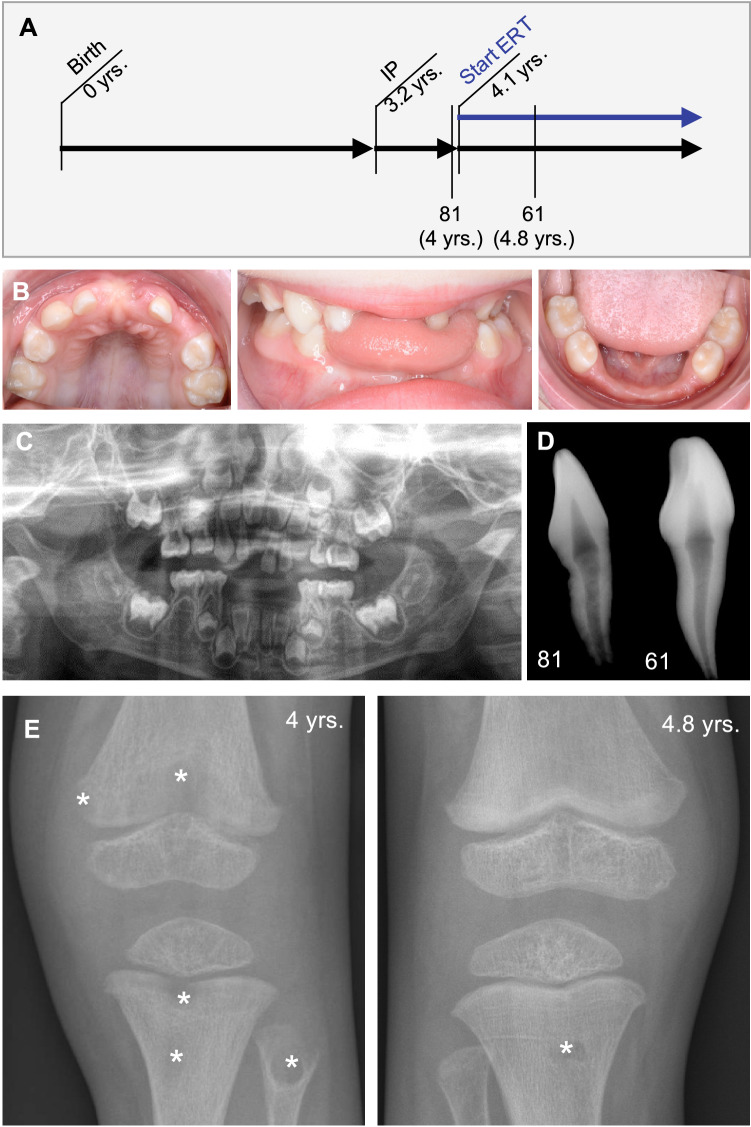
Dental and osteologic status of the male patient 2 before and during ERT with asfotase alfa. A Timeline of patient 2 with initial presentation (IP), start of ERT with asfotase alfa (ERT), and exfoliation of both analyzed teeth. B Clinical presentation with intraoral photographic images showing normal development of alveolar bone and premature loss of deciduous teeth without signs of periodontal disease or major root resorption at IP. C Radiological presentation at IP indicating a rotation and misalignment of the remaining teeth. D Radiographic images of lost teeth before (tooth 81) and under (tooth 61) ERT indicating a normal pulp and an intact root with minor signs of resorption only before ERT. E On the left panel, radiographic images of left knee before ERT with lesions (*), distended distal metaphases indicating delayed growth. On the right panel, right knee (following 8 months of asfotase alfa treatment) showing an improved bone microstructure. Usage of images were approved by the parents of the patient with written consent
